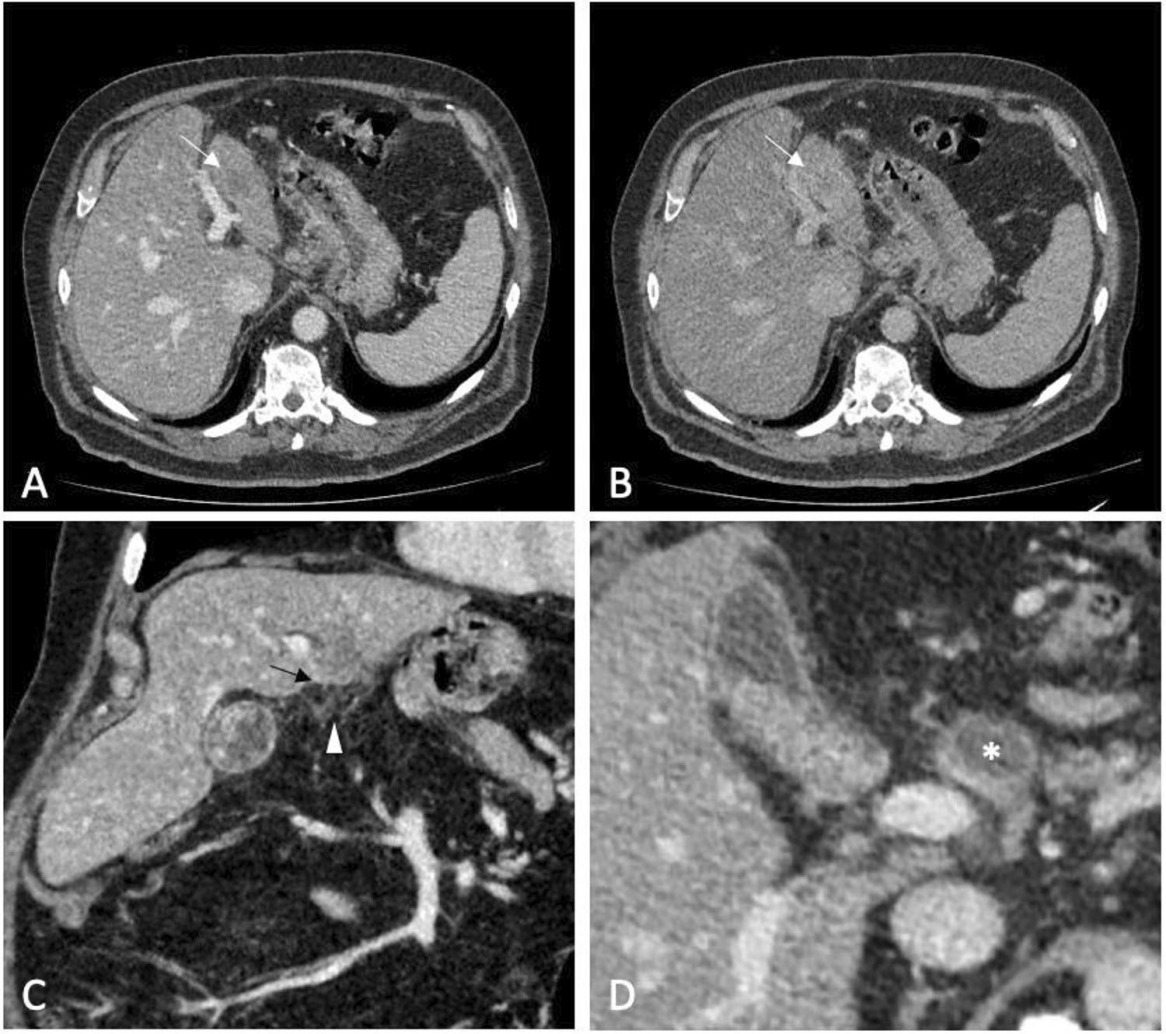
Axial contrast-enhanced CT images in portal (A) and equilibrium (B) phases showing a lesion in the III hepatic segment with progressive and delayed enhancement (white arrows). Minimal retraction of the capsule (black arrow) and perihepatic adipose tissue inhomogeneity (arrowhead) are visible in coronal view (C). Axial contrast-enhanced CT image in portal phase (D) also shows a hepatic hilar lymphadenopathy adjacent to the hepatic artery and characterized by a large central necrotic component (asterisk)
